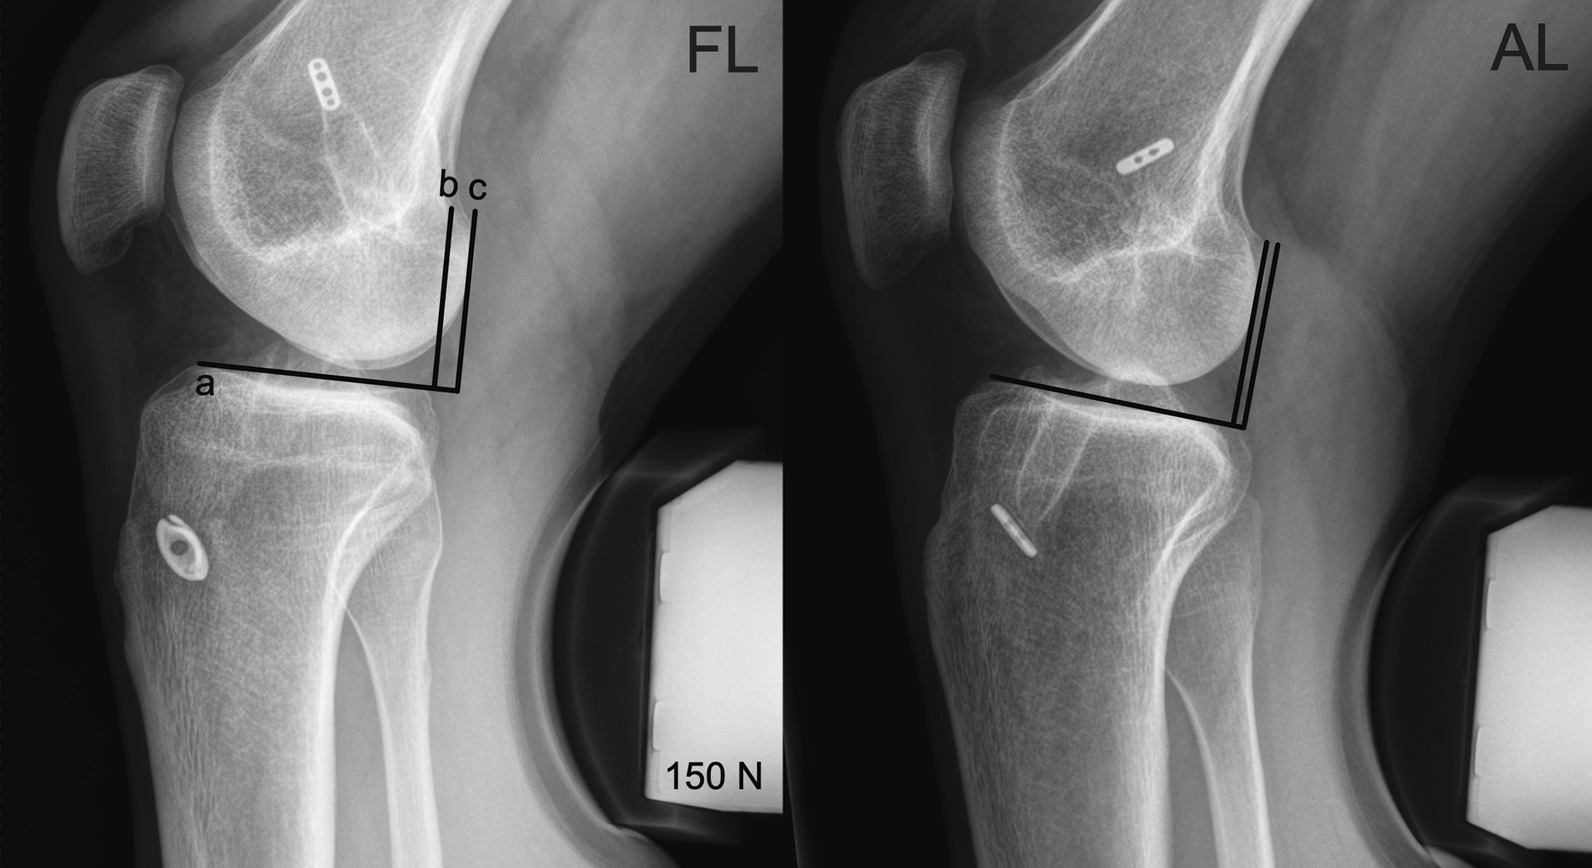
Anterior laxity measurement – Sagittal stress x-ray with the Telos device. (FL) 31-year-old male patient after FL ACL reconstruction. a Tangent to the medial tibial plateau, b line through the posterior edge of the femoral condyles and perpendicular to the tangent, c line through the posterior edge of the medial tibial plateau and perpendicular to the tangent. (AL) 26-year-old male patient after AL ACL reconstruction
